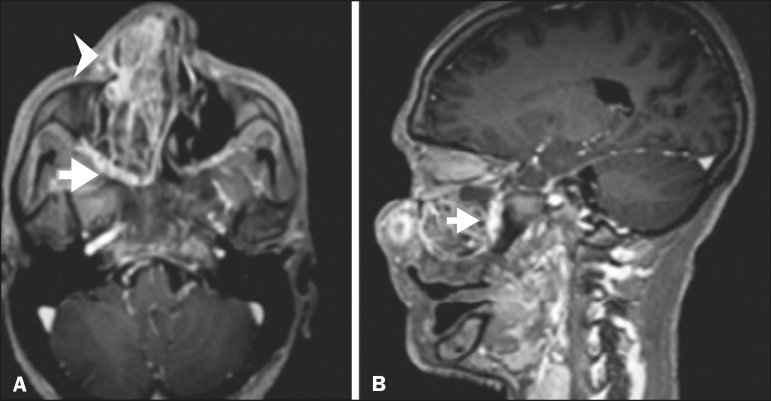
Gadolinium contrast-enhanced T1-weighted MRI scans of a 66-year-old
